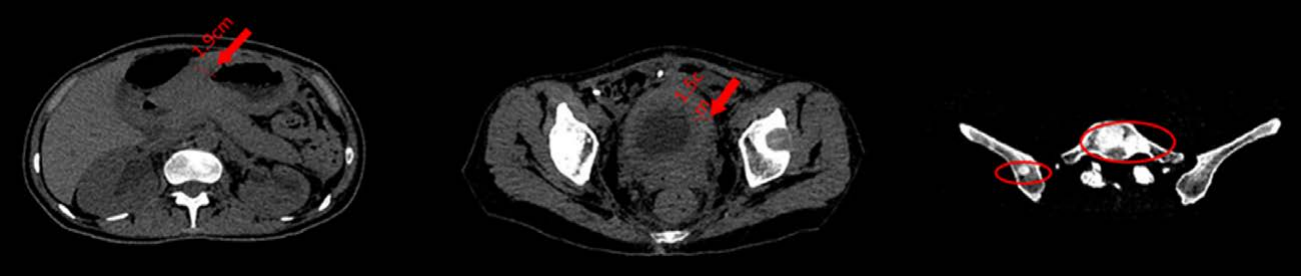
Changes in visible lesions on CT scans in November 2021. CT = computed tomography.
